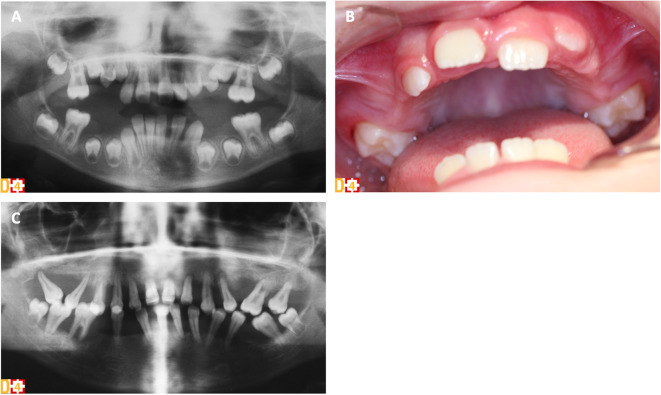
Clinical and radiological oral phenotype of two sisters with PLS syndrome (D[4]/Phenodent database (www.phenodent.org), Reference Center for Oral and Dental Rare Diseases, University Hospital, Strasbourg). (A,B) Premature tooth loss of the primary teeth in the 6-year-old patient. (A) Panoramic radiograph showing alveolar bone loss around the teeth, in particular at the mesial aspect of right permanent mandibular and maxillary first molars. (B) Intra-oral view showing the absence of all primary teeth. Absence of inflammation in the edentulous areas but presence of gingival inflammation around the erupting permanent maxillary first molars despite the perfect control of dental plaque. (C) Several periodontitis in the 16-year-old patient. Panoramic radiograph showing generalized severe vertical alveolar bone loss around all permanent teeth: typical radiological aspect of “floating” teeth.
